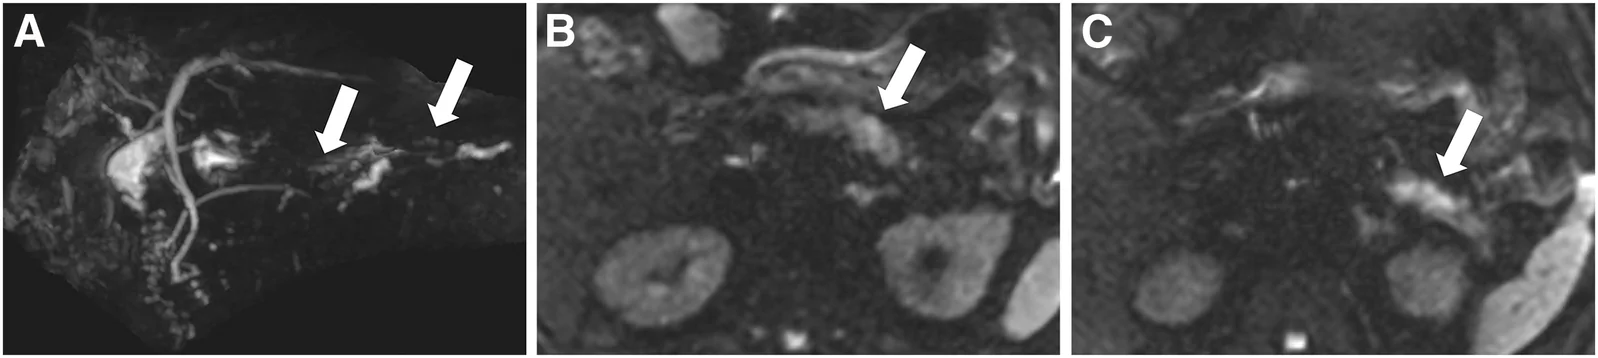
MRI findings. (A) Two main pancreatic duct strictures identified on magnetic resonance cholangiopancreatography. (B) Diffusion restriction at the site of the main pancreatic duct stricture in the pancreatic body. (C) Diffusion restriction at the site of the main pancreatic duct stricture in the pancreatic tail.
